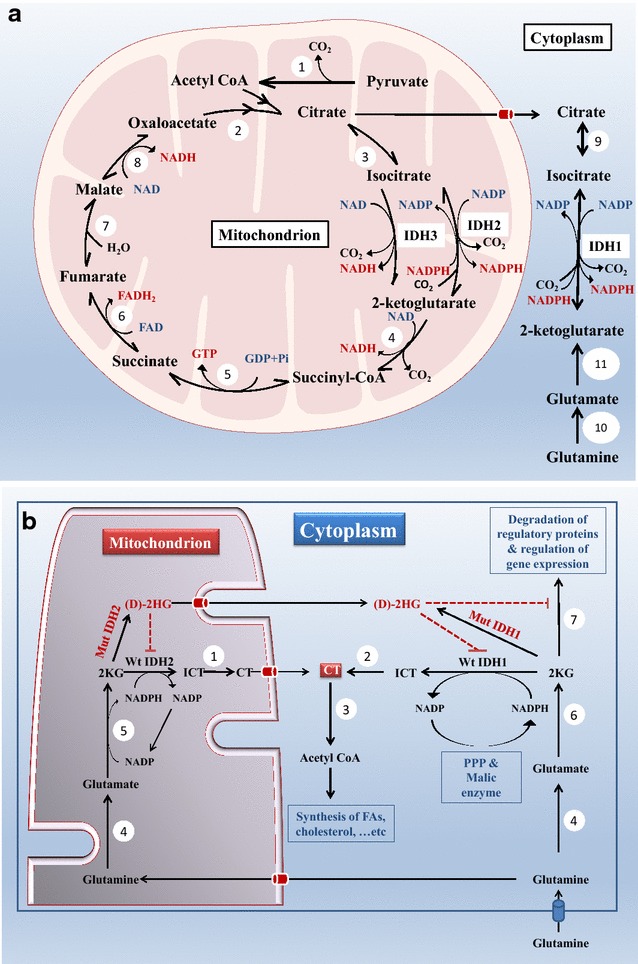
a The subcellular localization of the three isocitrate dehydrogenase isoforms and their roles in normal cellular metabolism. *IDH* isocitrate dehydrogenase, *1* pyruvate dehydrogenase, *2* citrate synthase, *3* mitochondrial aconitase, *4* 2-ketoglutarate dehydrogenase, *5* succinyl CoA synthetase, *6* succinate dehydrogenase, *7* fumarate hydratase, *8* malate dehydrogenase, *9* cytosolic aconitase, *10* glutaminase, *11* glutamate transaminase. b The roles of wild type and mutant isocitrate dehydrogenases 1 and 2 in cellular metabolism. *CT* citrate, *(D)-2HG* (d) 2-hydroxy glutarate, *FAs* fatty acids, *ICT* isocitrate, *IDH* isocitrate dehydrogenase, *Mut* mutant, *PPP* pentose phosphate pathway, *Wt* wild type, *2KG* 2-keto glutarate, *1* mitochondrial aconitase, *2* cytosolic aconitase, *3* citrate lyase, *4* glutaminase, *5* glutamate dehydrogenase, *6* glutamate transaminase, *7* 2-ketoglutarate dependent dioxygenases. The *dotted red line* indicates inhibition
